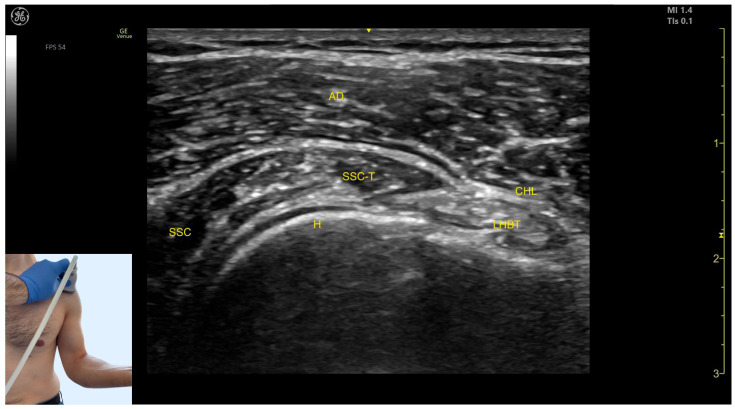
Ultrasound anatomy of the subscapularis (SSC) anterior view with key landmarks: AD—anterior deltoid; SSC—subscapularis; SSC-T—subscapularis tendon; H—humerus; LHBT—long head bicep tendon; and CHL—coracohumeral ligament.
